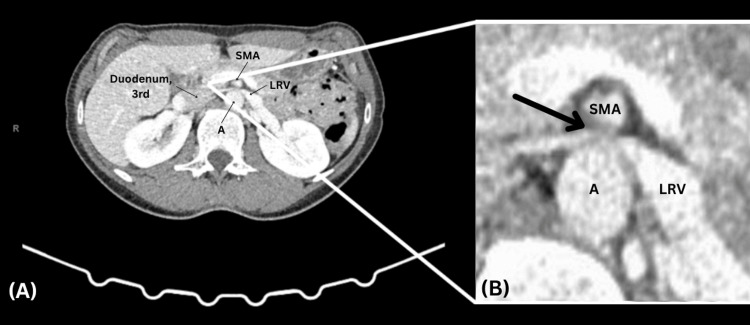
Contrast-enhanced CT demonstrating compression of the duodenum and LRV. Contrast-enhanced CT in axial projection. (A) Compression of the third portion of the duodenum consistent with superior mesenteric artery (SMA) syndrome, with a reduced aortomesenteric angle. (B) Narrowing of the left renal vein (LRV) compressed between the aorta (labeled 'A') and the SMA, with a characteristic beak sign (arrow).
